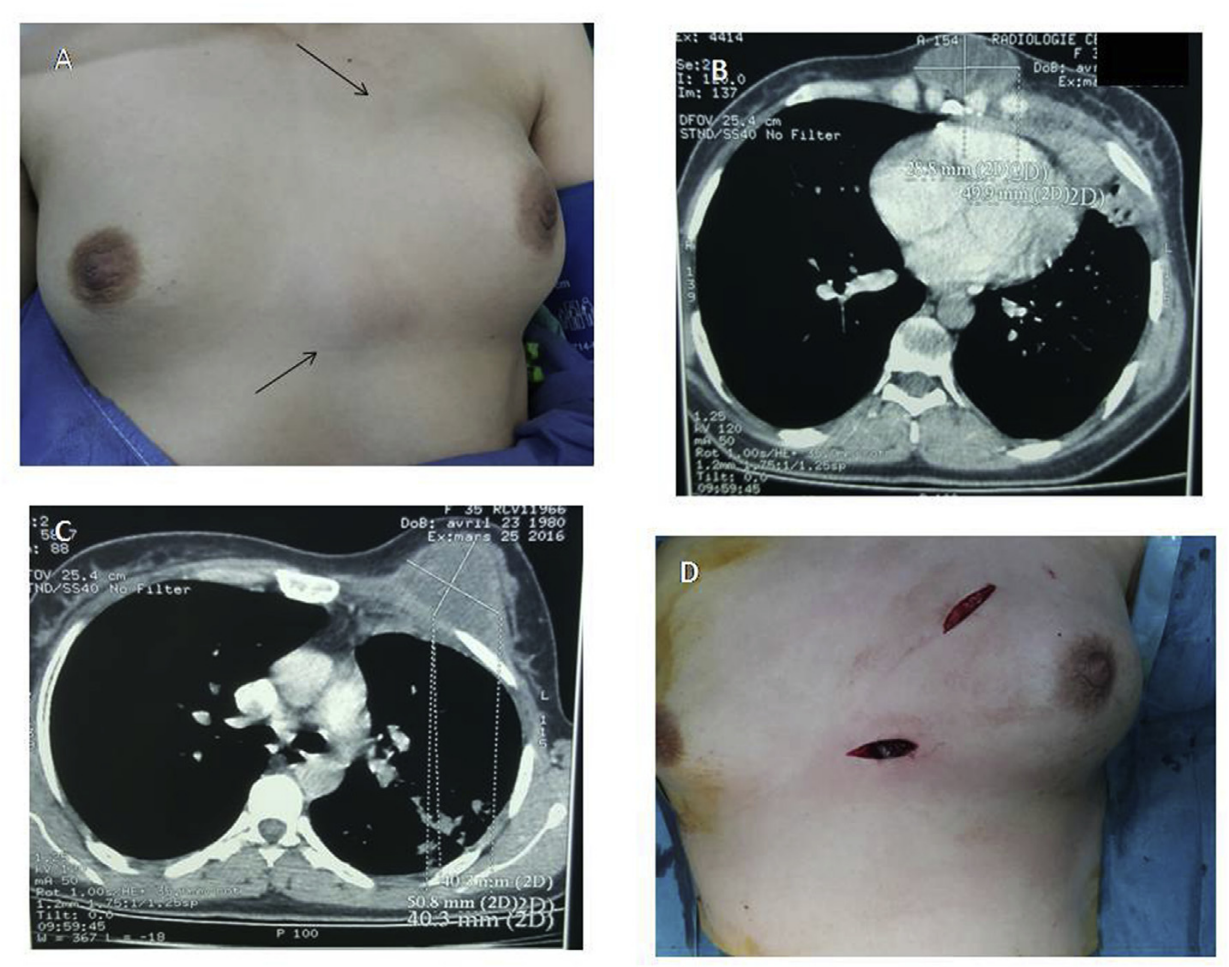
A: Two swelling anterior chest wall in the left side just up to the breast and the second next to the xyphoid appendix. B and C: Chest computed tomography showing two anterior abscesses (median and left side). D: Double incision for drainage the collections and wide debridement (Patient no: 9).
